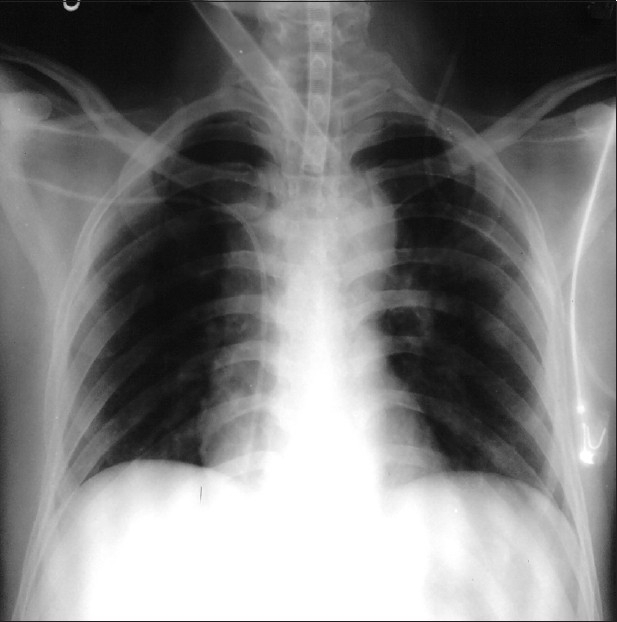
Chest X-ray with catheter *in situ*
